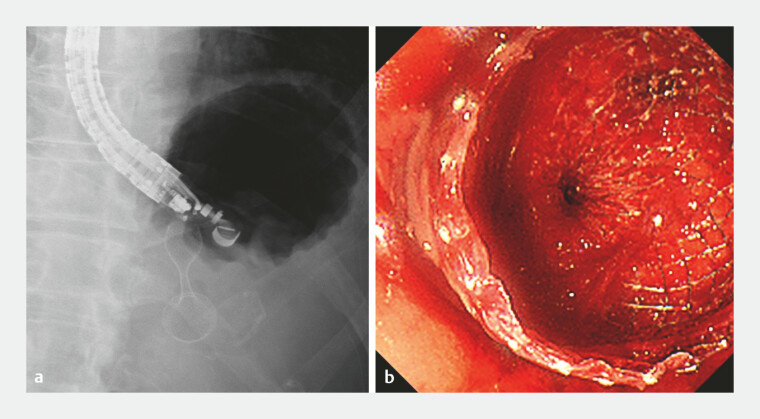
A radiographic image showing lumen-apposing metal stent placement (
